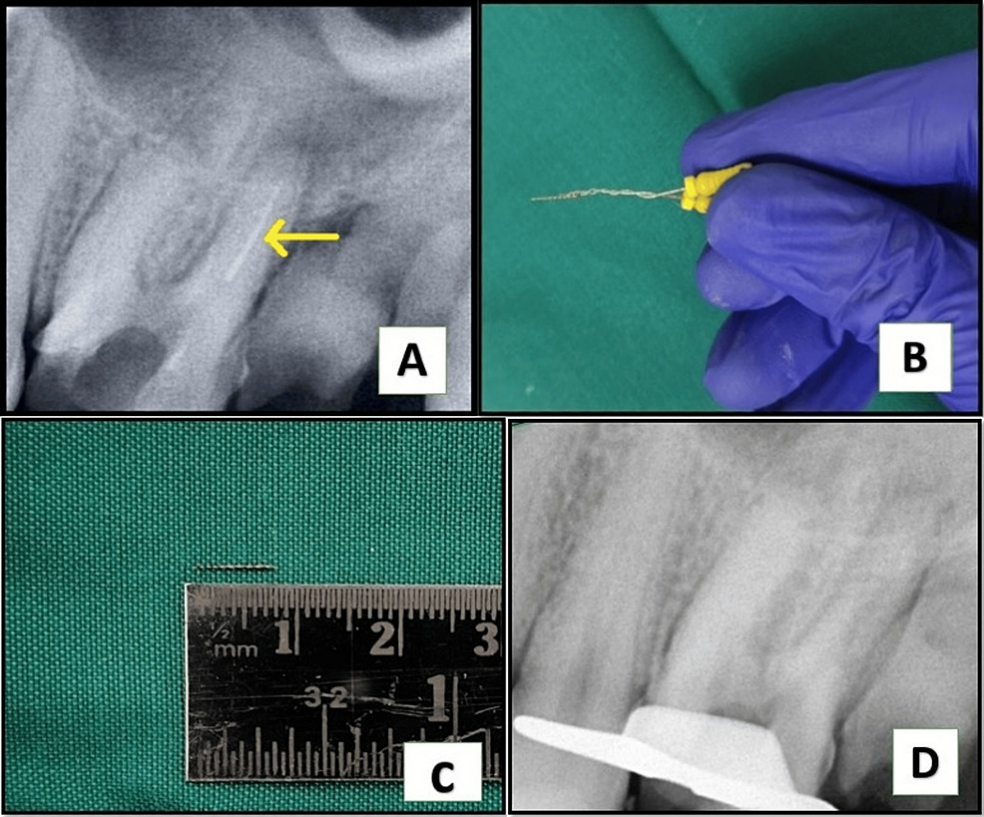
(A) Radiograph showing the separated instrument in the distobuccal canal. (B) Retrieval of the instrument seen clinically with the braiding technique. (C) Measurement of the separated file. (D) Post-retrieval radiograph.
